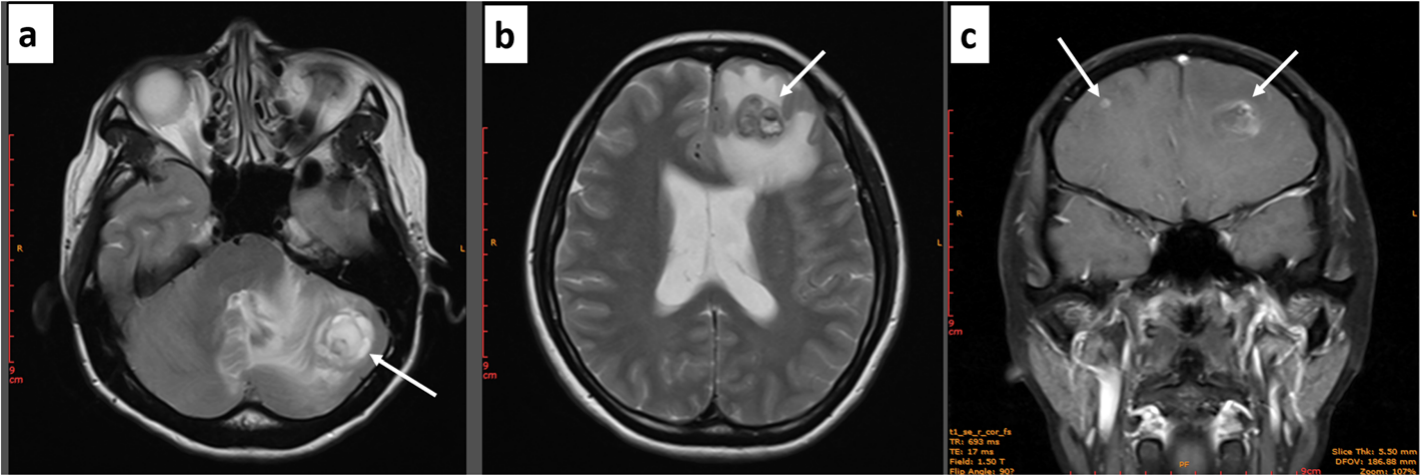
Multiple metastases (white arrows) in the brain as scanned by MRI. (a) metastases in the left cerebellar hemisphere in the horizontal plane; (b) metastases in the left frontal lobe in the horizontal plane; (c) metastases in the bilateral frontal lobe in the coronal plane
